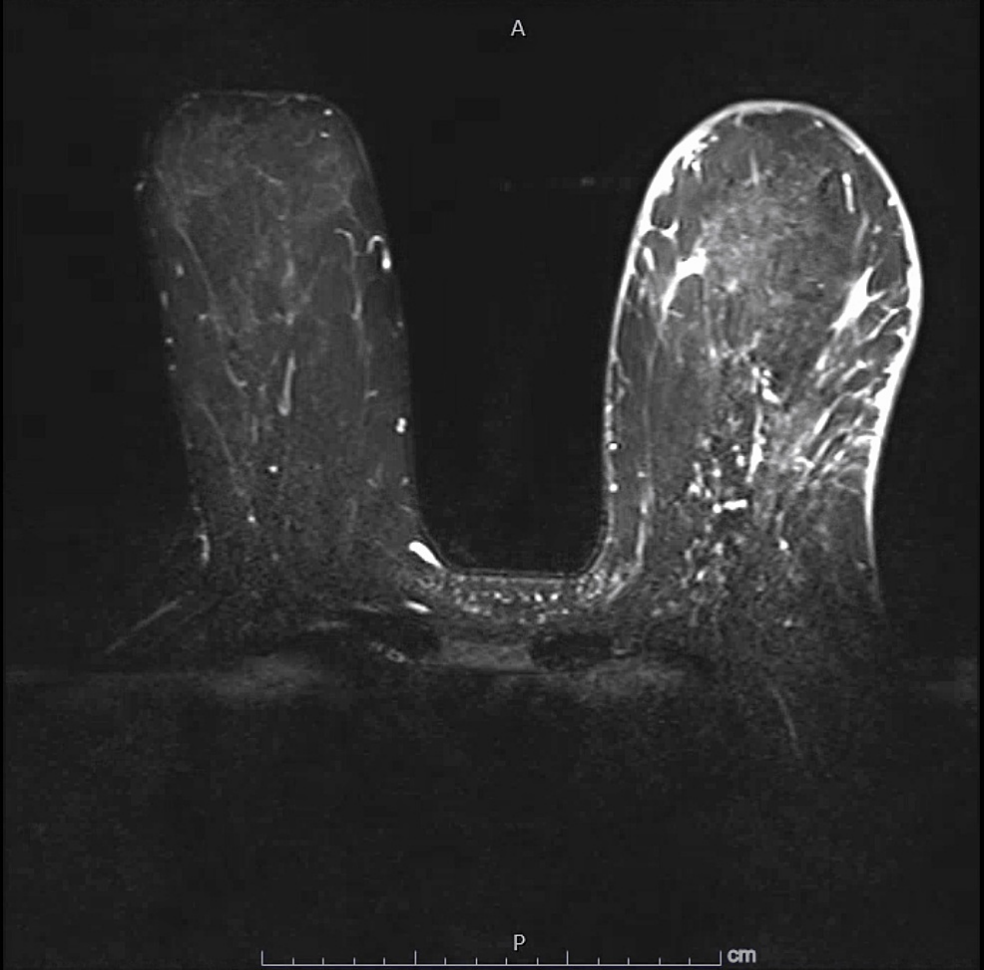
Breast MRI again revealed significant skin thickening and vascularity of the left breast
